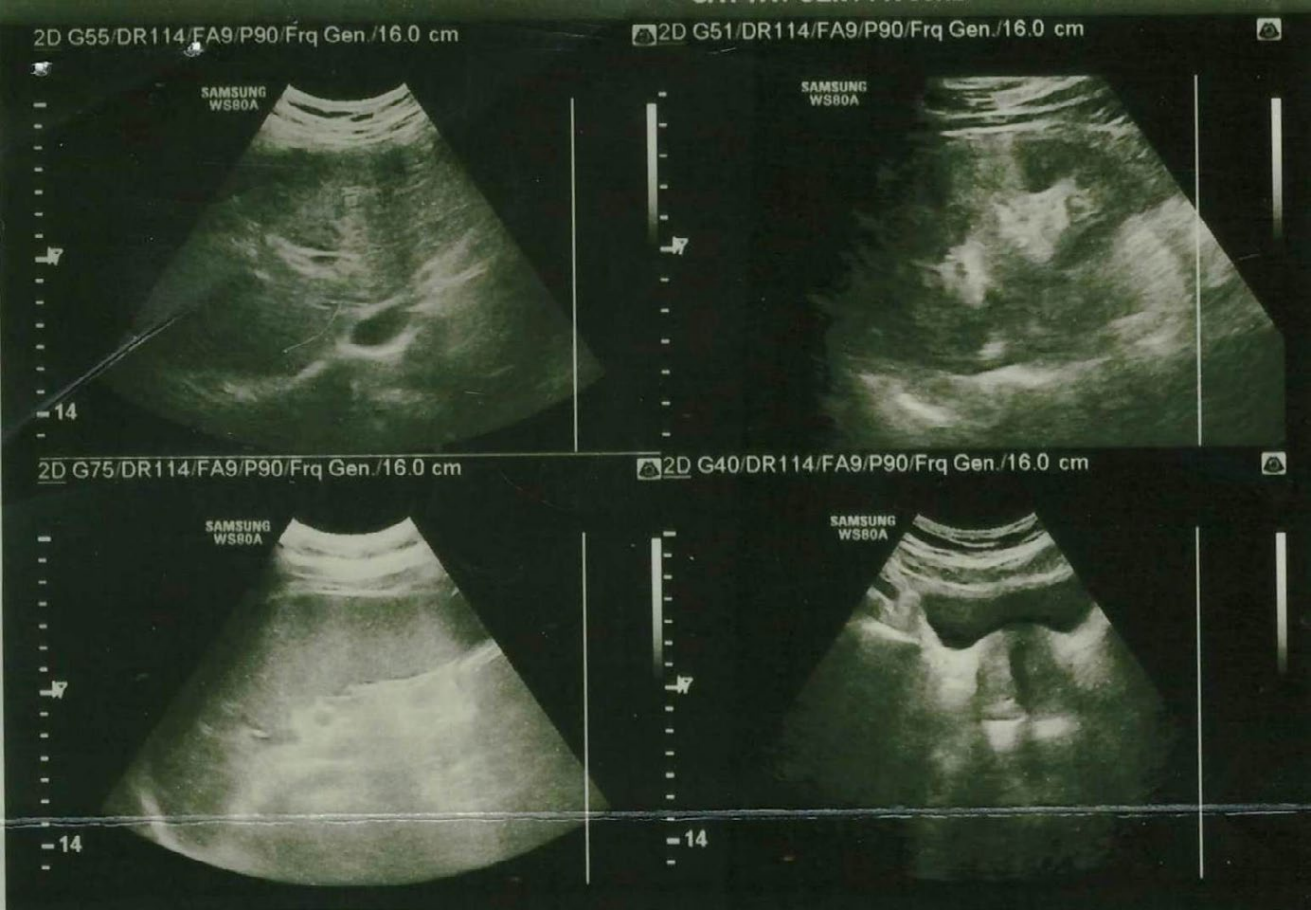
Ultrasonography of liver and normal portal vein diameter
